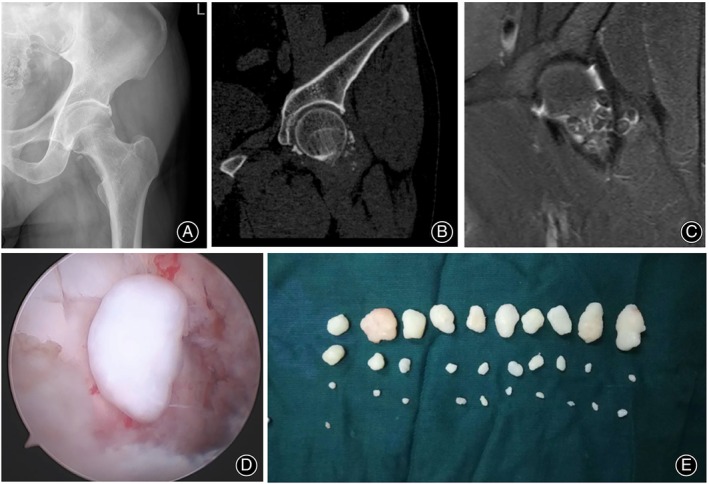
The size of loss bodies varies greatly in PT‐1. Radiographic appearance: (A) x‐ray, (B) computed tomography (CT) coronal reconstruction image, and (C) magnetic resonance (MR) T2‐weighted image. (D) Arthroscopic view. (E) Multiple loose bodies in different sizes.
